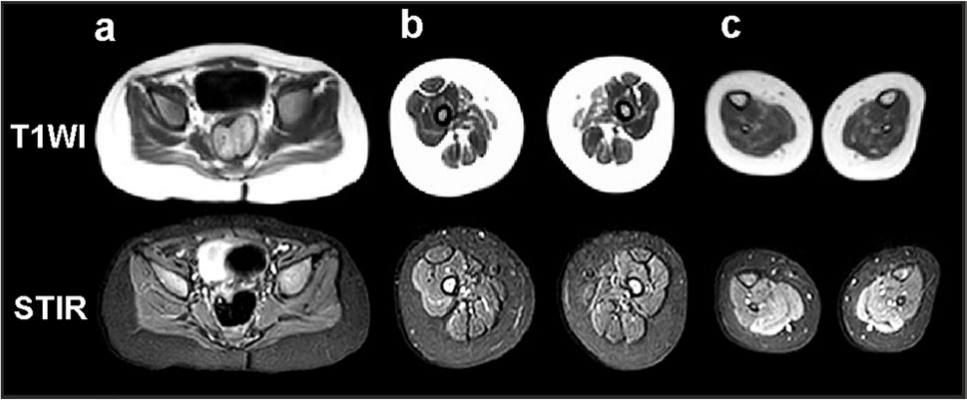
MRI (T1, STIR) of the pelvic muscles (a), thigh muscles (b), and legs (c)
